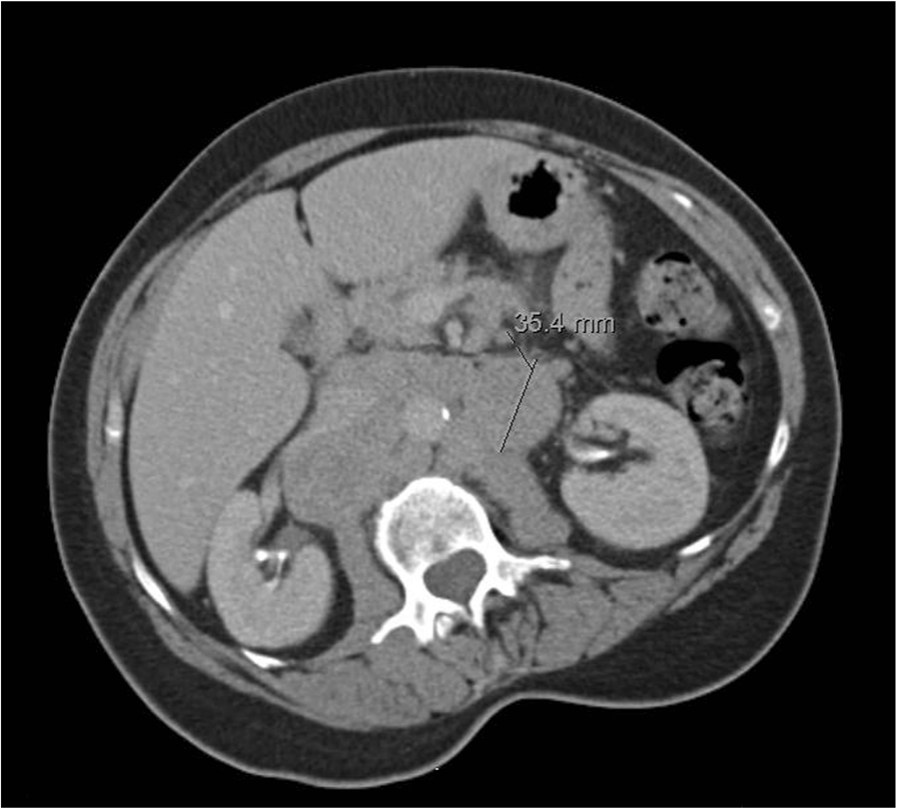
CT scan. Extensive bulky retroperitoneal lymphadenopathy (lymph node of 35.4 mm is marked on the picture).
